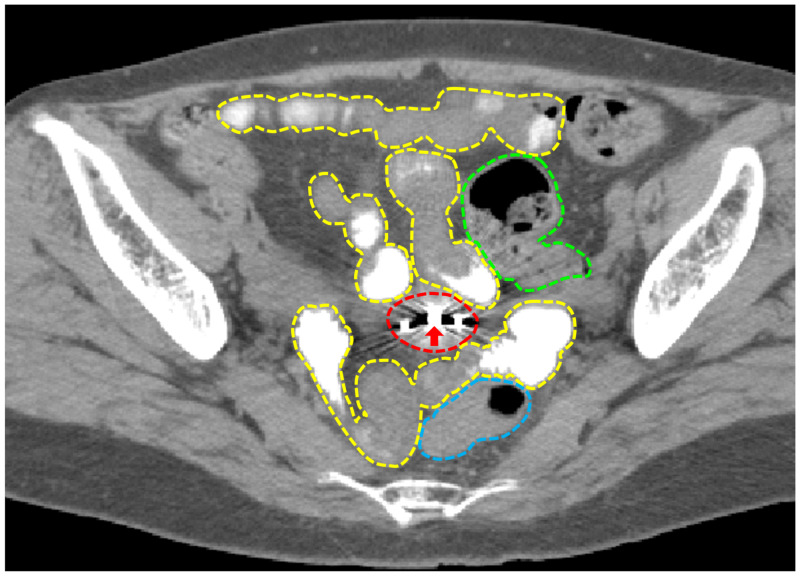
CT images used for treatment planning of brachytherapy with oral gastrografin pretreatment. The dashed yellow, green, blue, and red lines show the small intestine, the sigmoid colon, the rectum, and the high-risk clinical target volume, respectively. The arrow shows the tandem applicator inserted in the endometrial cavity.
